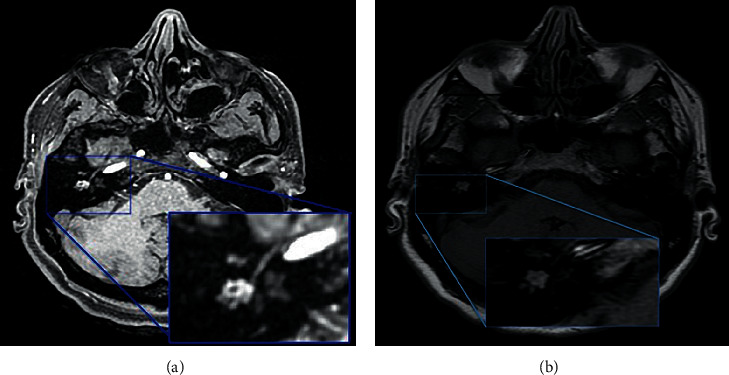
Axial magnetic resonance imaging (MRI) scans of the middle ear. (a) T1 fat-saturated MRI revealed tissue in the middle ear. (b) T1 diffusion-weighted imaging did not show a restriction of diffusion in the tissue, as is typical of cholesteatoma.
